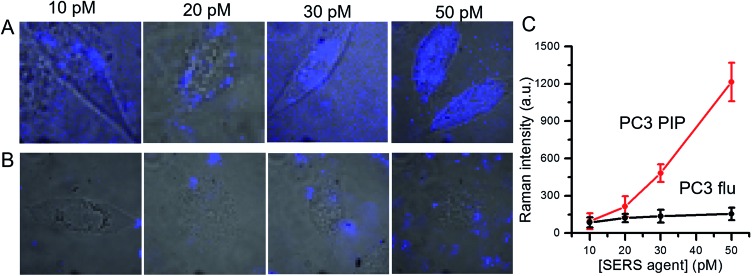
Dose-dependent SERS images of (A) PSMA+ PC3 PIP and (B) PSMA– PC3 flu cells following incubation in various concentrations (10, 20, 30 and 50 pM) of SERS agents. The SERS images were overlaid with bright-field images to aid understanding of the spatial localization of the agents. (C) Concentration-dependent SERS intensity determined by averaging the SERS intensity over the pixels on a single cell. The SERS images were constructed on the basis of the integrated intensity of the 1340 cm^–1^ Raman band.
